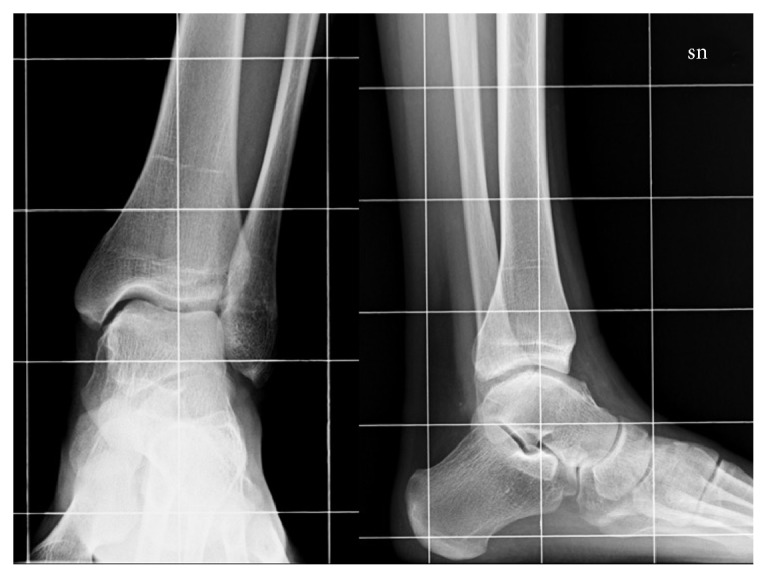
Five-year follow-up X-rays without signs of recurrence or arthritis.
